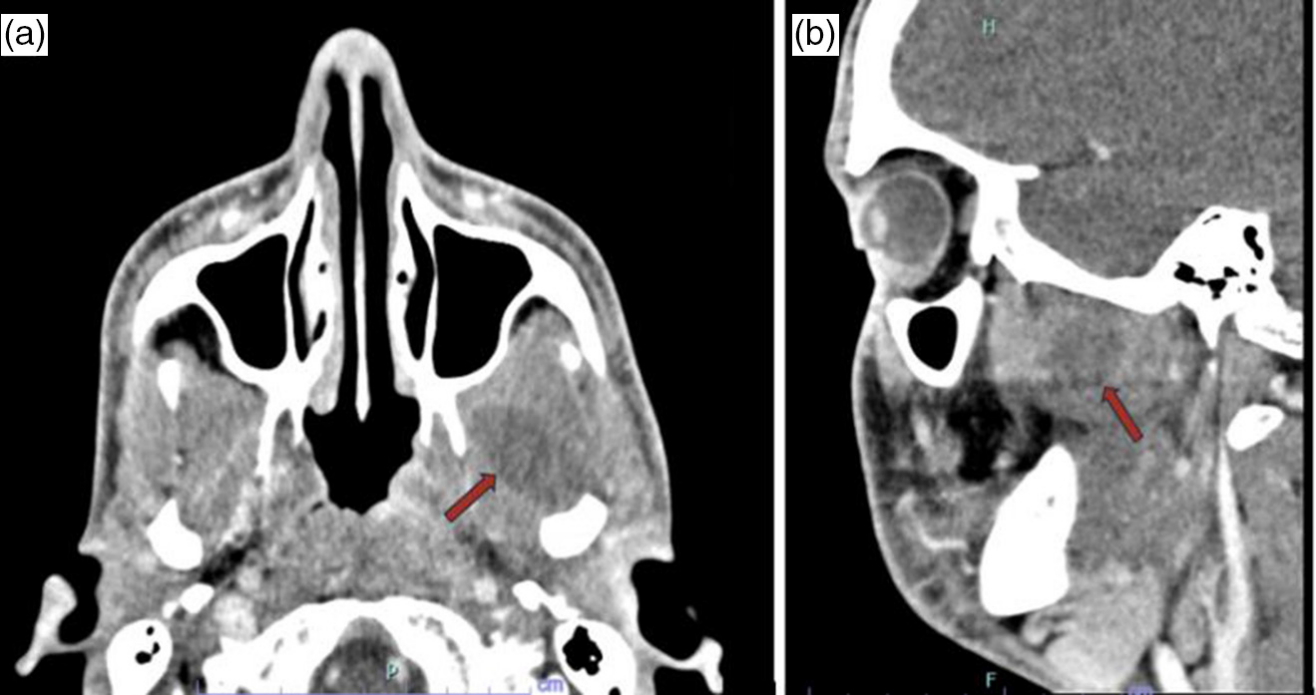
Rim-enhancing lesion in the left pterygoid musculature. (a) Axial and sagittal (b) view of maxillofacial CT with contrast demonstrating a left pterygoid musculature abscess measuring 2.7×1.6×1.6 cm with surrounding oedema in the medial and lateral pterygoids.
